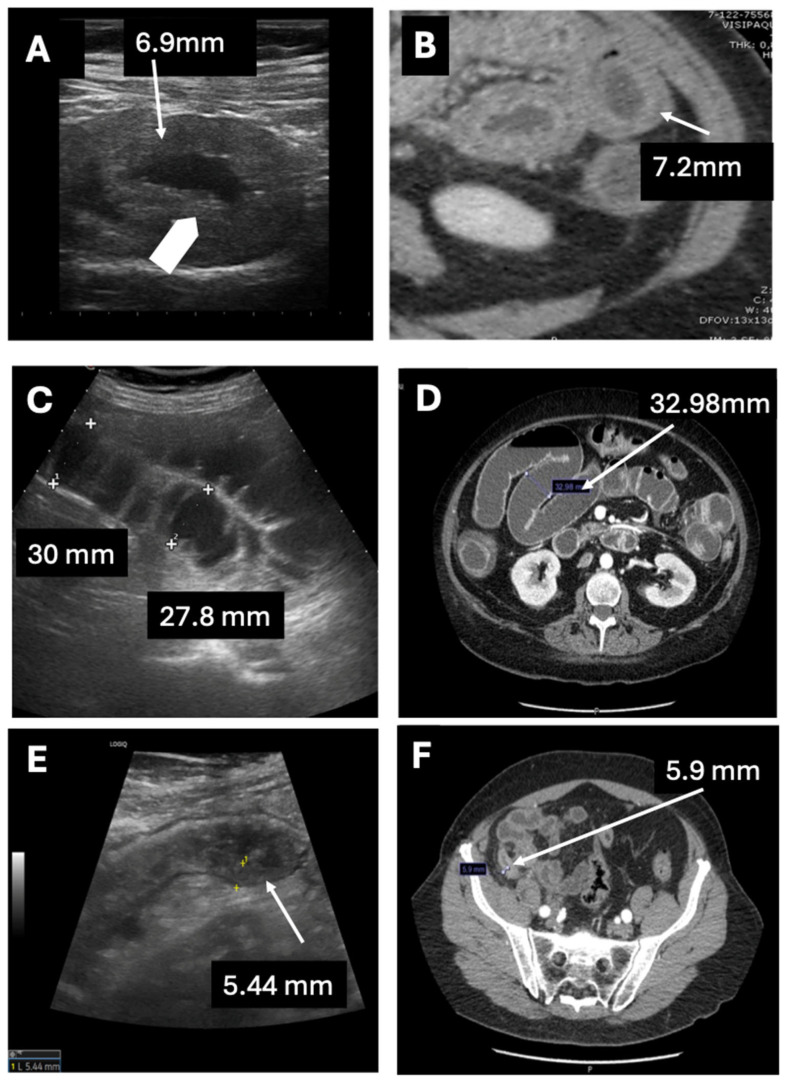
Comparison between CT and BS-US in the diagnosis of NEC (panel A and B), abdominal distention (panel C and D) and appendicitis (panel E and F). (A) BS-US showing NEC with BWT 6.9 mm; (B) CT showing BWT 7.22 mm; white arrowheads show a microbubble of air within injured bowel wall visible with both BS-US and CT. (C) BS-US showing intestinal distension with fluid filled content (30 mm and 27.8 mm); (D) same images in CT (32.98 mm); (E) BS-US showing acute appendicitis (5.44 mm); (F) same image with CT (BWT 5.9 mm).
